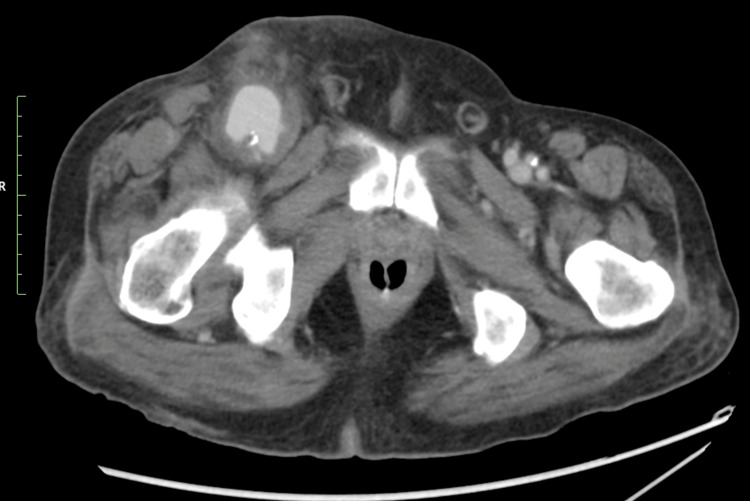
Contrast-enhanced computed tomography image of a pseudo arterial aneurysm Contrast-enhanced computed tomography demonstrating a pseudoaneurysm in the right inguinal region (approximately 46 × 41 mm), with surrounding inflammatory changes.
